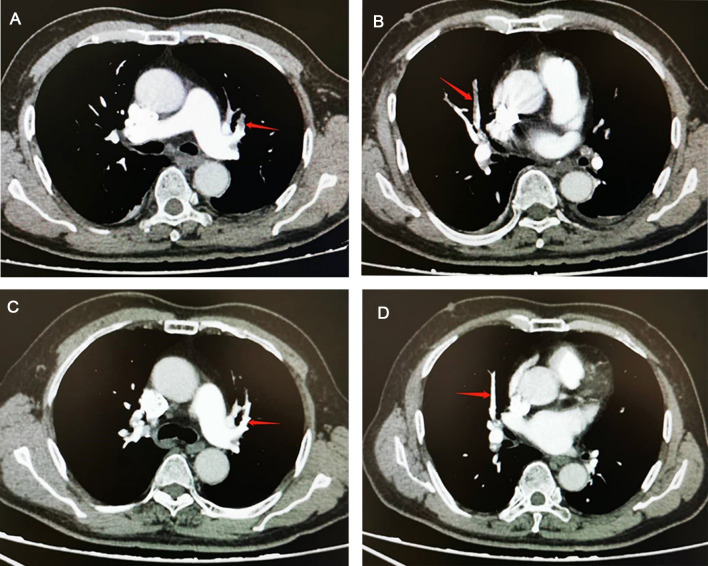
Comparison of computed tomographic pulmonary arteriography (CTPA) images before and after treatment in a pulmonary embolism (PE) patient. (A,B) CTPA shows filling defects in the left upper pulmonary artery and right upper pulmonary artery (red arrow). (C,D), CTPA shows that filling defects in the left upper pulmonary artery and right upper pulmonary artery have disappeared after administering anticoagulants for one month (red arrow).
